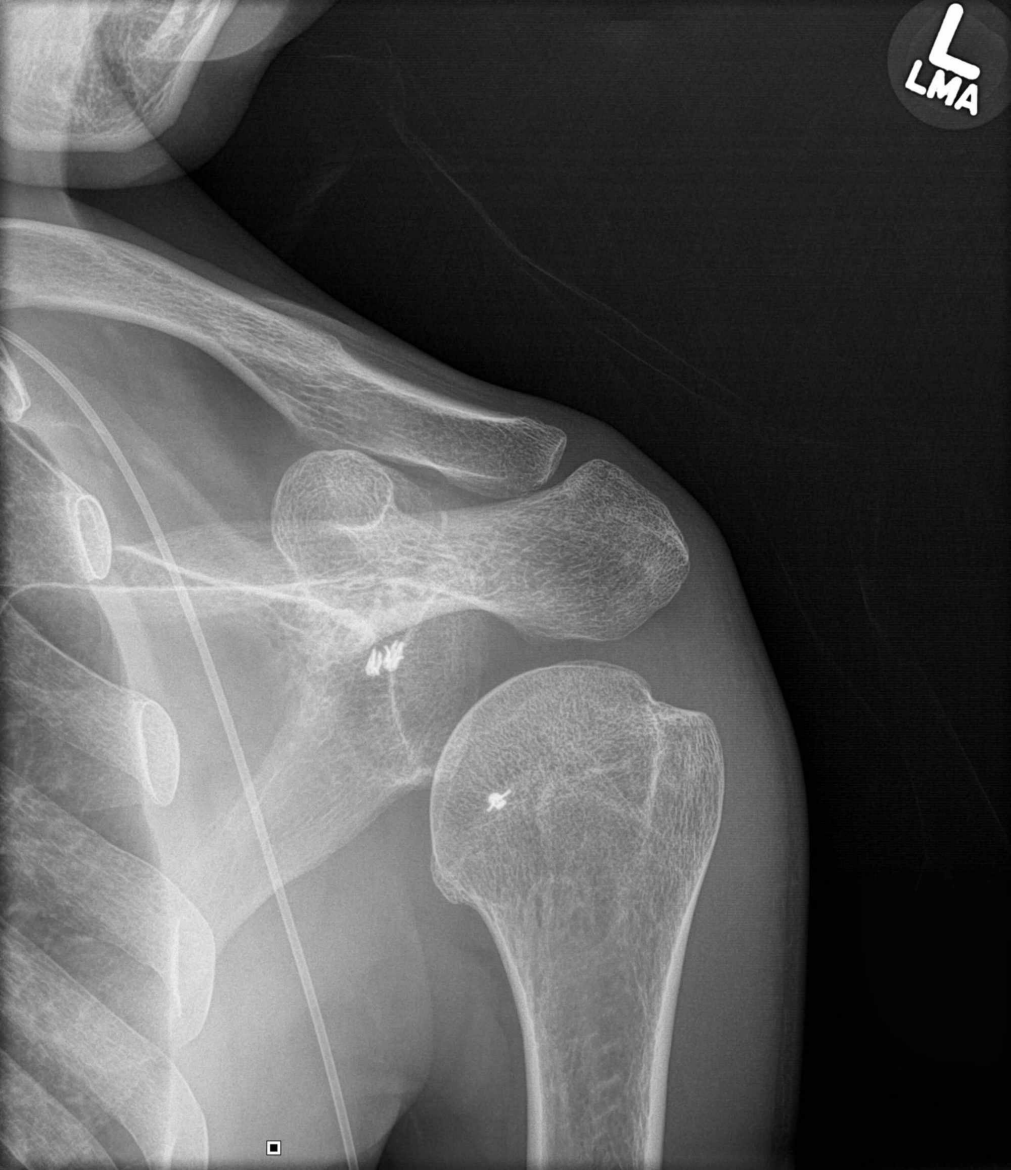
Grashey view radiograph of the shoulder showing posteroinferior subluxation of the humeral head.
